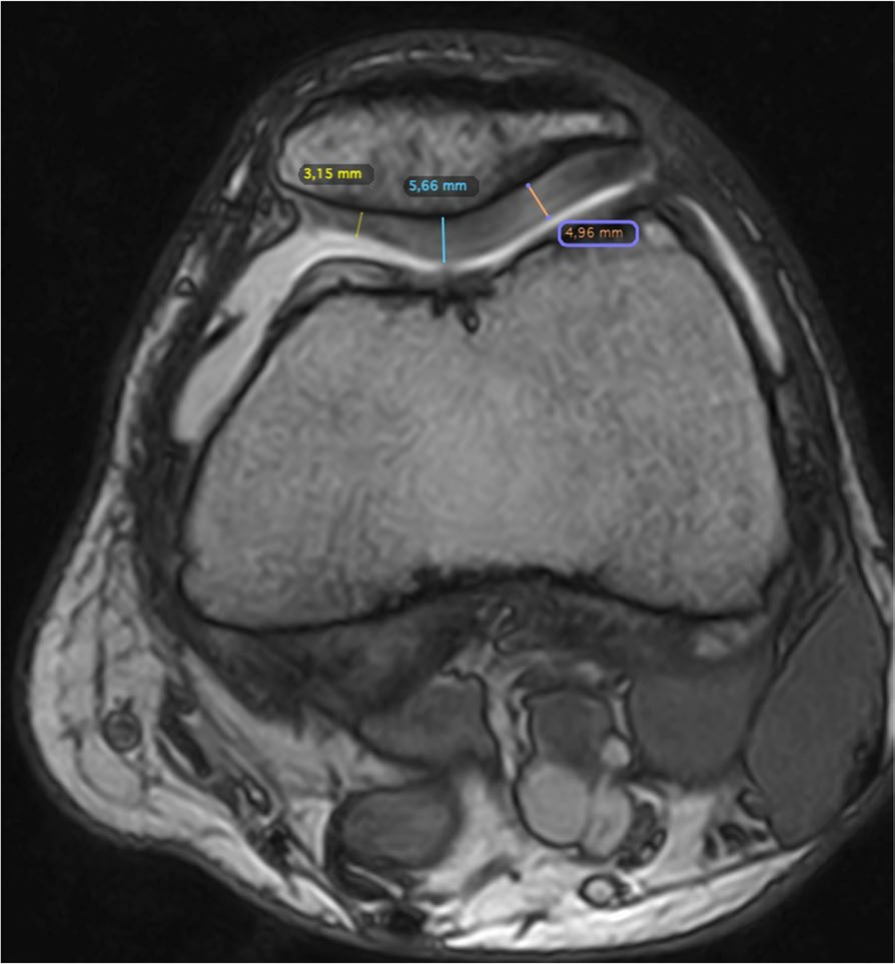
MRI cross-section with reference points for the patellar cartilage measurement
